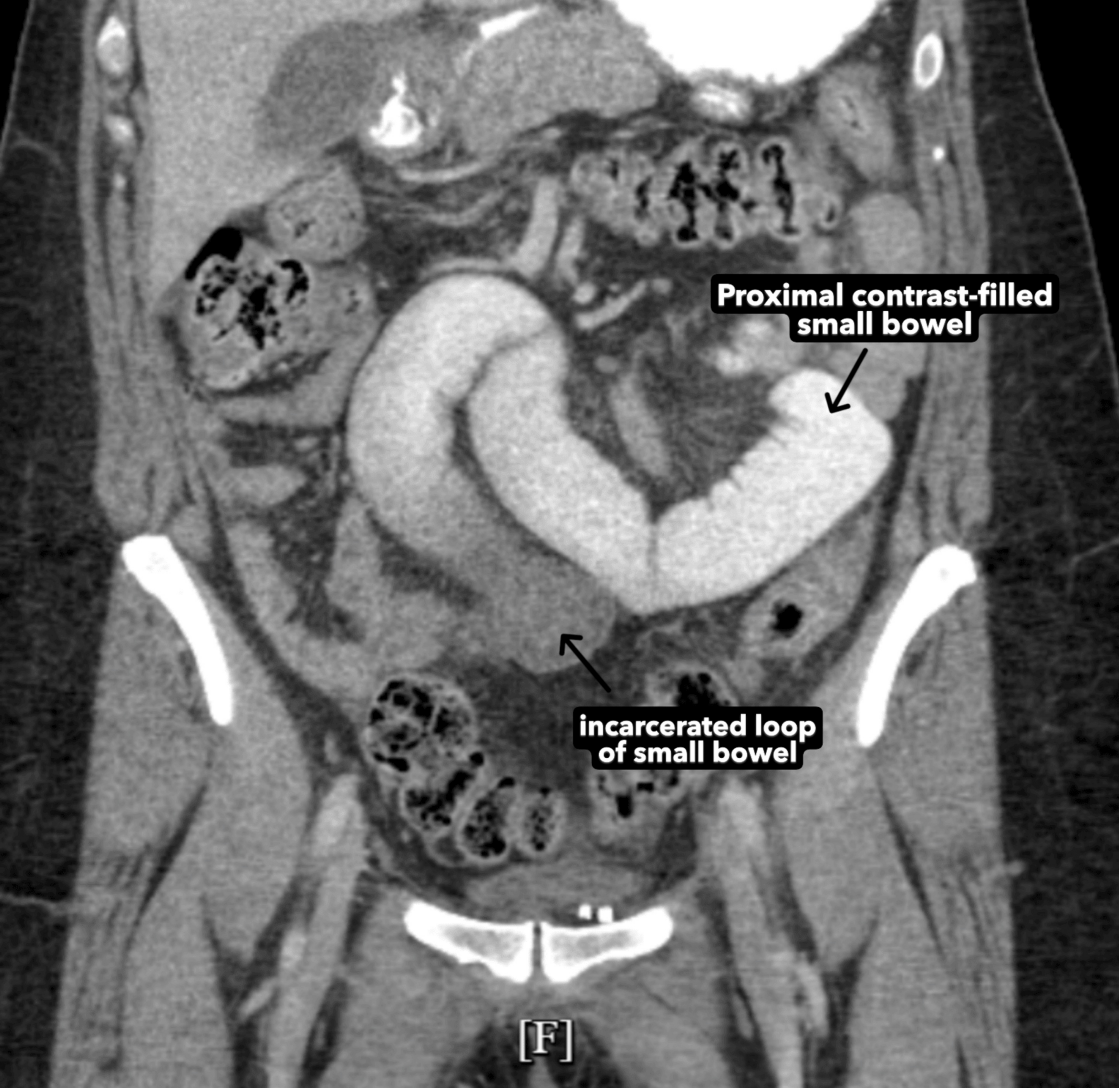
CT scan findings of an incarcerated bowel loop in the lower midline abdomen (coronal view) This coronal slide of the CT with oral contrast shows a dilated, contrast-filled portion of the small bowel that is proximal to the site of the hernia. The proximal dilation indicates obstruction of the small bowel at the site of the hernia. The lack of oral contrast within the incarcerated loop is indicative of obstruction at the site of the hernia.
